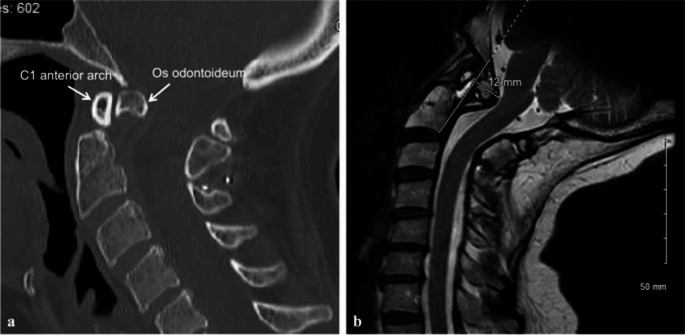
(A) Non-contrast cervical CT imaging demonstrated os odontoideum with retrolisthesis of C1 on C2 and dislodged wiring from previous surgery. (B) MRI demonstrated a Grabb-Oakes line measuring 12 mm with a ventral mass effect on the brainstem and spinal cord
